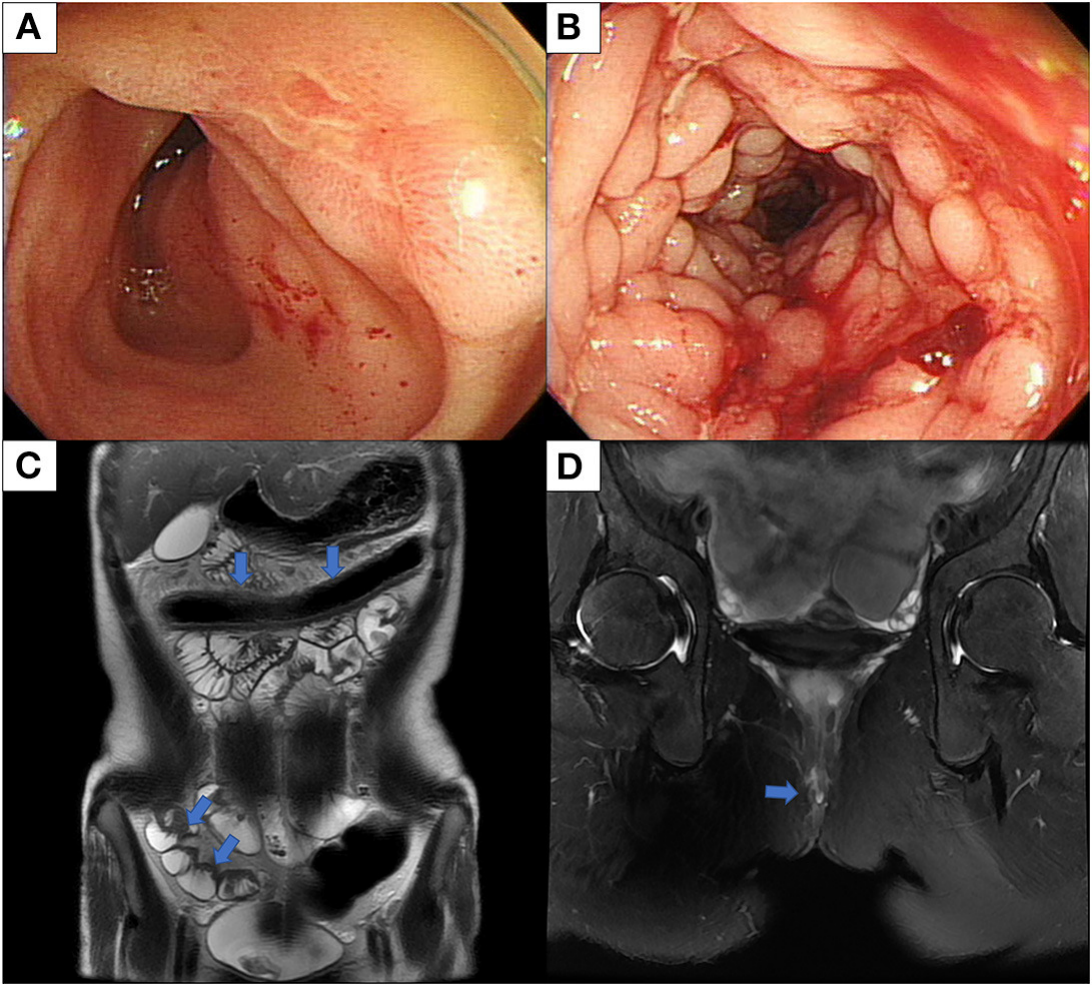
Ileocolonoscopy and MRI conducted at diagnosis of Crohn's disease. (A) Ileocolonoscopy revealed an ulcer in the terminal ileum, and (B) linear deep ulcers with cobblestoning in the transverse colon. (C) MRE shows multifocal asymmetric bowel wall thickening involving the transverse colon, terminal ileum, and jejunum and luminal narrowing in the transverse colon with diffusion restriction and enhancement. (D) MRI of the pelvis shows two intersphincteric perianal fistulas. MRI, magnetic resonance imaging; MRE, magnetic resonance enterography.
